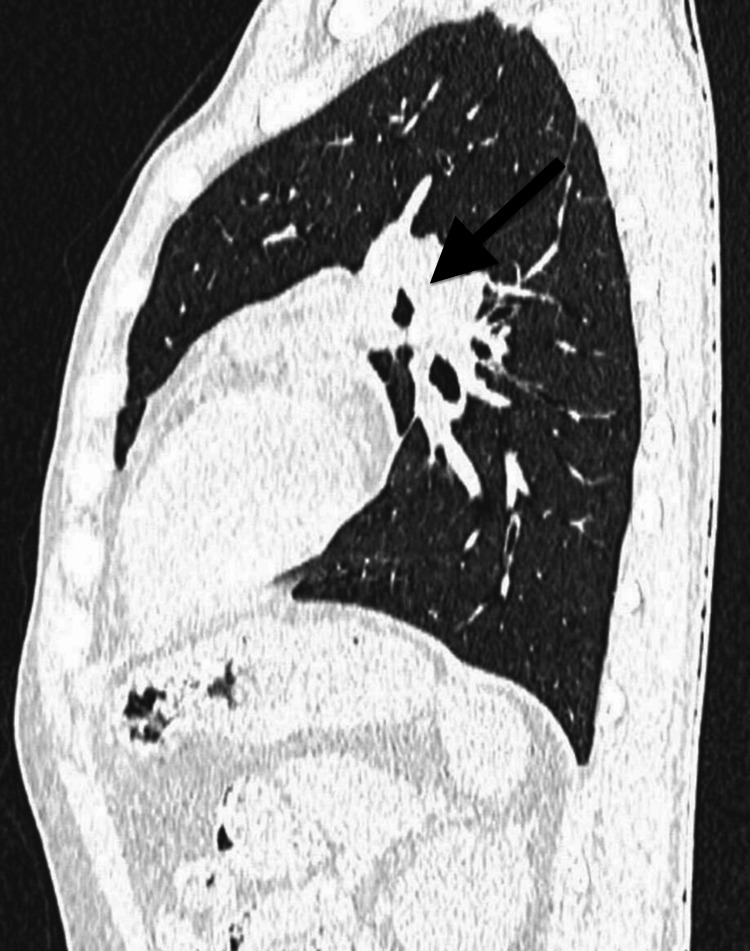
Computed tomogram of the chest sagittal view (lung window) showed conglomerated nodes forming a ring-like configuration around the airway, strikingly resembling a "Medu Vada" (doughnut)
